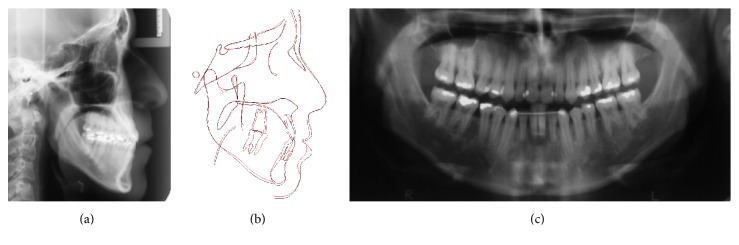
(a, b) Movements of the upper and lower incisors can be noted in the cephalometric X-ray; (c) Upper removable appliance and a 3-3 lower retainer used for retention.
